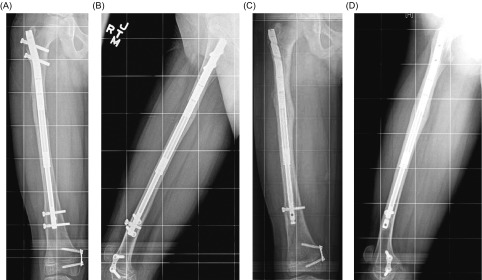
Clinical case with partial union. AP (A) and lateral (B) view radiographs of the right femur show partial union. After injection of bone marrow aspirate concentrate (platelet-rich plasma) into the partial union site and nail dynamization by removing the proximal locking screws, AP (C) and lateral (D) view radiographs showed complete healing. Figure used with permission (© 2016, Rubin Institute for Advanced Orthopedics, Sinai Hospital of Baltimore).
